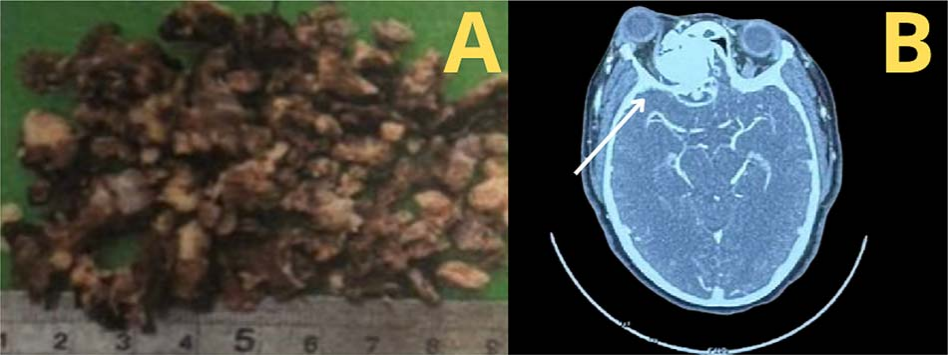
(A) Macroscopic examination of ossifying fibroma, (B) CT.
